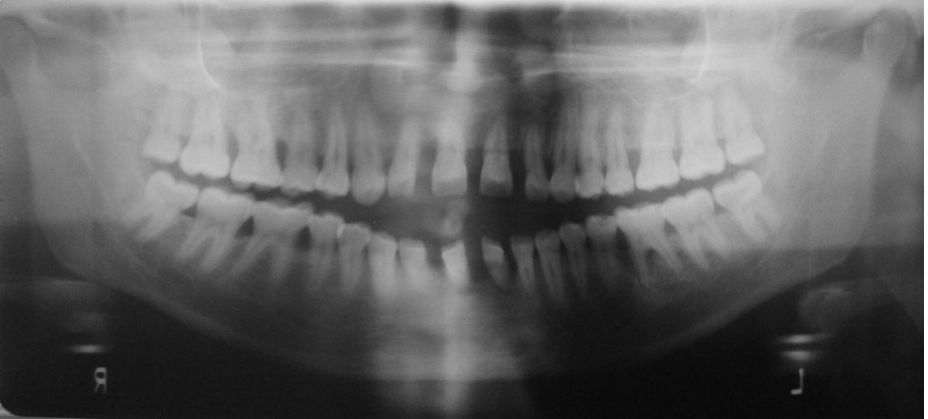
Orthopantomograph showed severe horizontal alveolar bone loss anteriorly, and vertical bone loss around 31 and 36.
